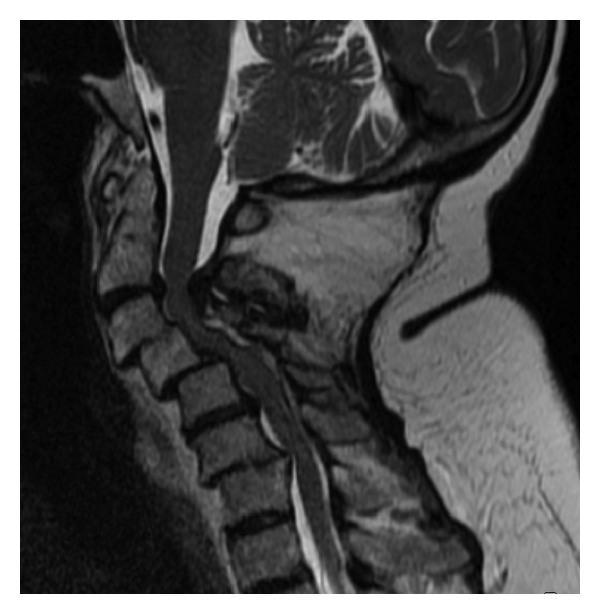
Sagittal MRI scan demonstrating the subaxial “staircase” deformity and significant posterior ligamentous hypertrophy contributing to cervical myelopathy.
